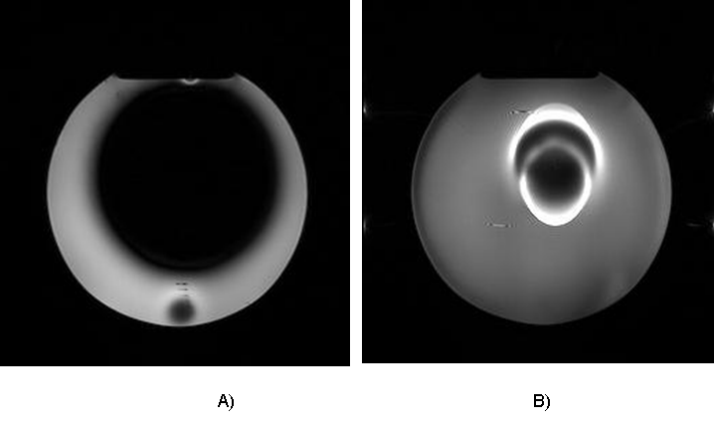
Artifact on MR image: The Polaris valve was placed in a water-filled container, with diameter equivalent to adult skull). A) Gradient echo image; B) T1 image.
